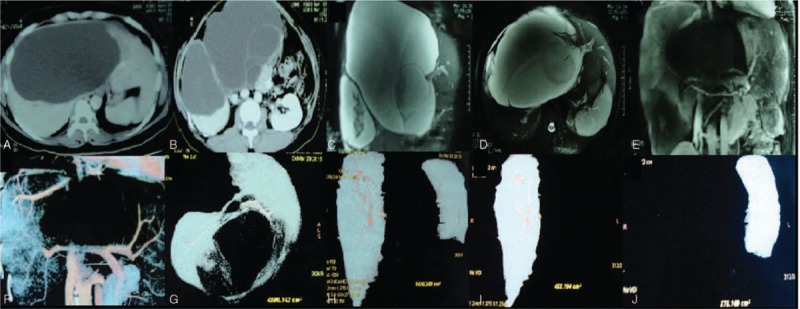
Imaging examination. (A, B) CT showed 2 masses (approximately 13.2 × 19.9 cm and 8.3 × 10.4 cm in size) of slightly low density. Septa were visible in the larger cyst, with enhancement of the cystic wall and septa and no significant enhancement inside the cyst. (C, D) MRCP showed dilation of the left intrahepatic bile ducts (possibly related to compression of the hilar bile ducts) and unclear extrahepatic bile ducts. (E) MRA showed compression and displacement of the hilar vessels, clear images of the trunk and branches of the portal vein, and compression and displacement of the hepatic veins. (F) CTA showed compression and displacement of the hilar vessels, clear images of the trunk and branches of the portal vein, a clear right hepatic vein (compressed and displaced), and an unclear left hepatic vein. (G, H, I, J) CT measurement showed that the overall liver volume was 4590 mL, and the FLV was 1030 mL: the FLV of the right liver was 455 mL, and the FLV of the left liver was 575 mL. CT = computed tomography, CTA = computed tomographic arteriography, FLV = future liver volume, MRA = magnetic resonance angiography.
